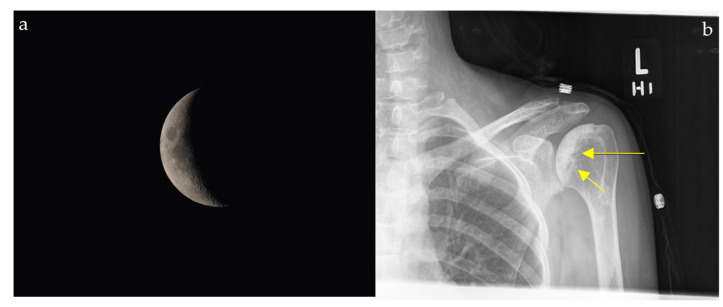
The appearance of a waxing gibbous, soon to be a crescent moon (a) resembling a linear cleft due to subchondral fracture in the setting of osteonecrosis seen on plain shoulder radiograph (yellow arrows) (b).
